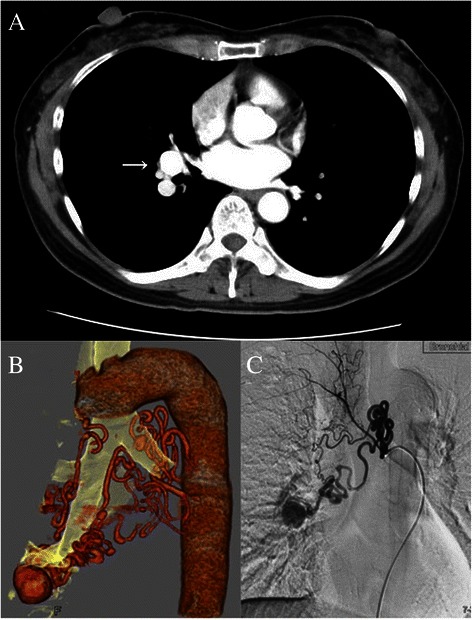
Preoperative CT scan showing the bronchial arterial aneurysm connected to the basal pulmonary artery (a), 3-D CT (b), and selective bronchial arteriography (c) revealing four tortuous feeding bronchial arteries and the saccular bronchial arterial aneurysm of the basal bronchus
